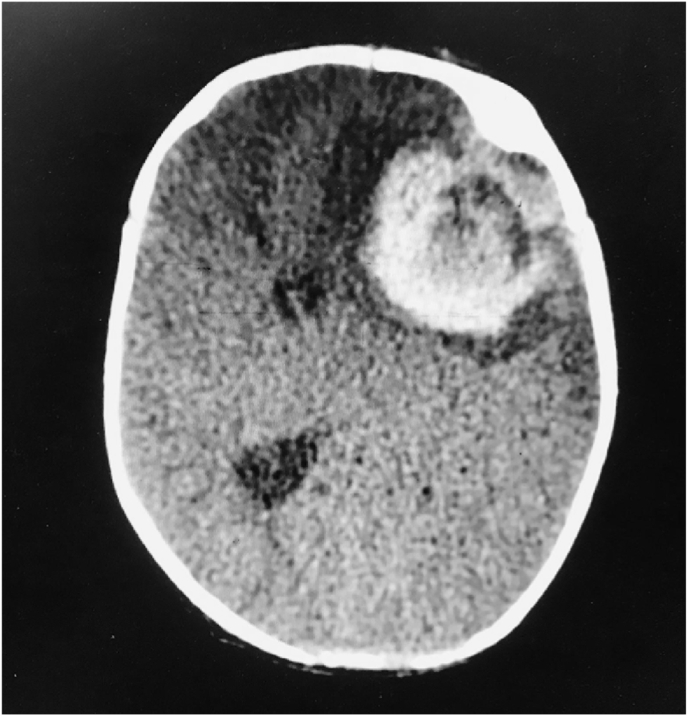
Head Computed Tomography scan demonstrates an acute hemorrhage in the left frontal lobe with associated vasogenic edema and severe left-to-right midline shift.
